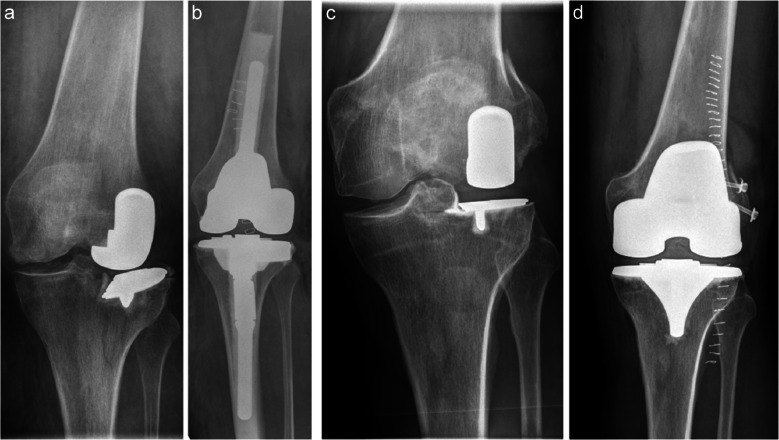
a A. p. radiograph showing a periprosthetic lateral tibia fracture after fixed-bearing lateral UKA (b) Postoperative a. p. radiograph after revision surgery with Vanguard 360 knee revision system with femoral (10 mm, lateral) and tibial (10 mm, lateral) augments (c) A. p. radiograph demonstrating a periprosthetic femoral fracture after fixed-bearing lateral UKA (d) Postoperative a. p. radiograph after revision to TKA and lag screw fixation of lateral femoral condyle
